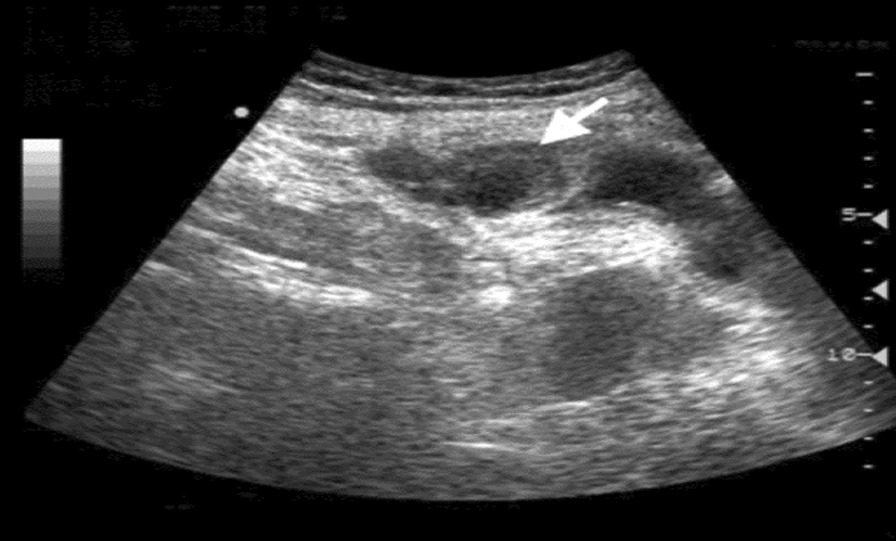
Abdominal ultrasonographic finding of a urachal cyst. Arrow indicating the urachal cyst
